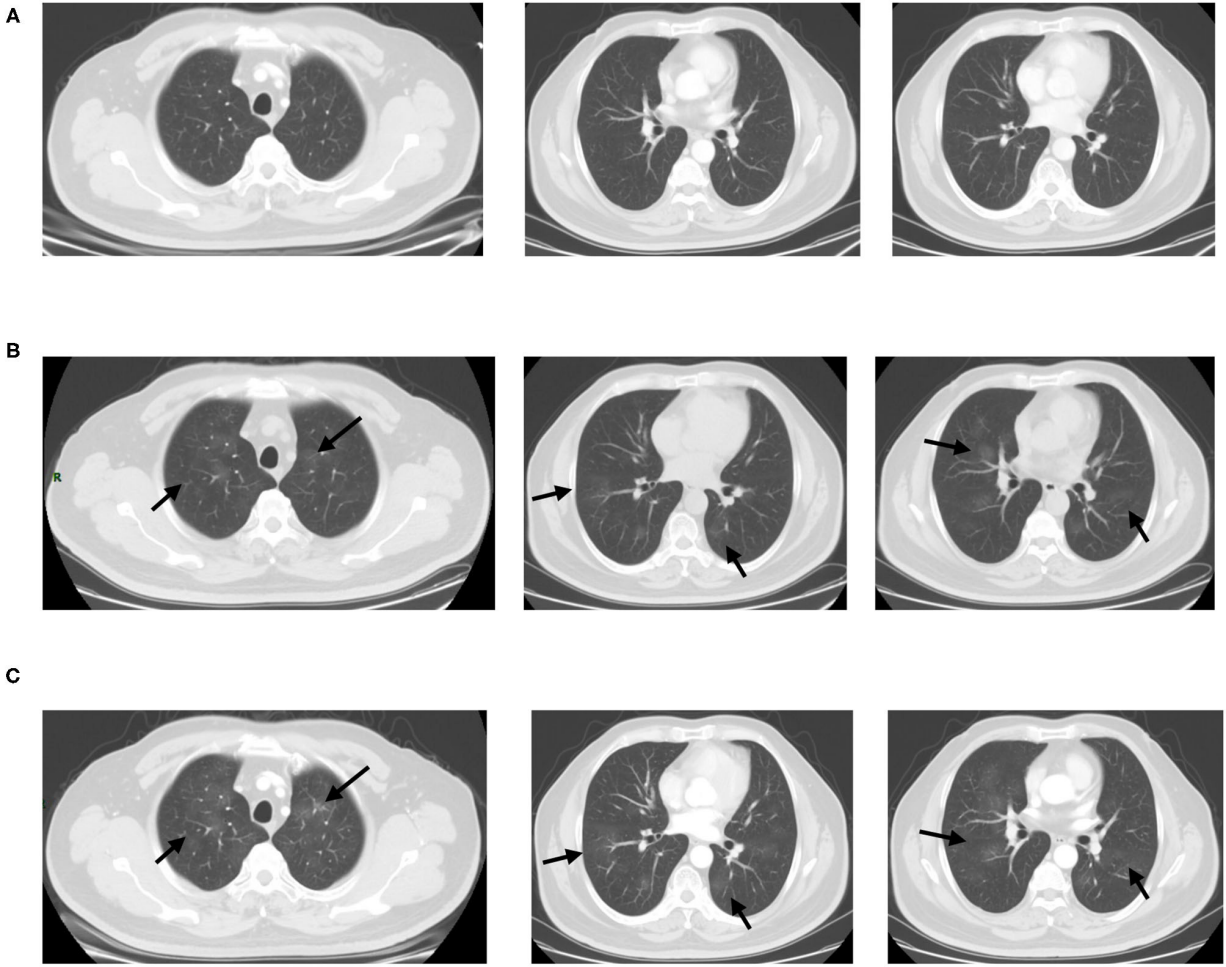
Fifty-six-year-old male with post-operation of ascending colon carcinoma history. Comparison with the prior CT which performed 1 month ago (A), screening CT scans of the chest (B) show bilateral multiple GGOs without vascular enlargement (arrows). Most of lesions were identified in the centrally distributed. The short-term follow-up CT scan (C) showed unchanged (arrows). The RT-PCR results of the patient was negative.
